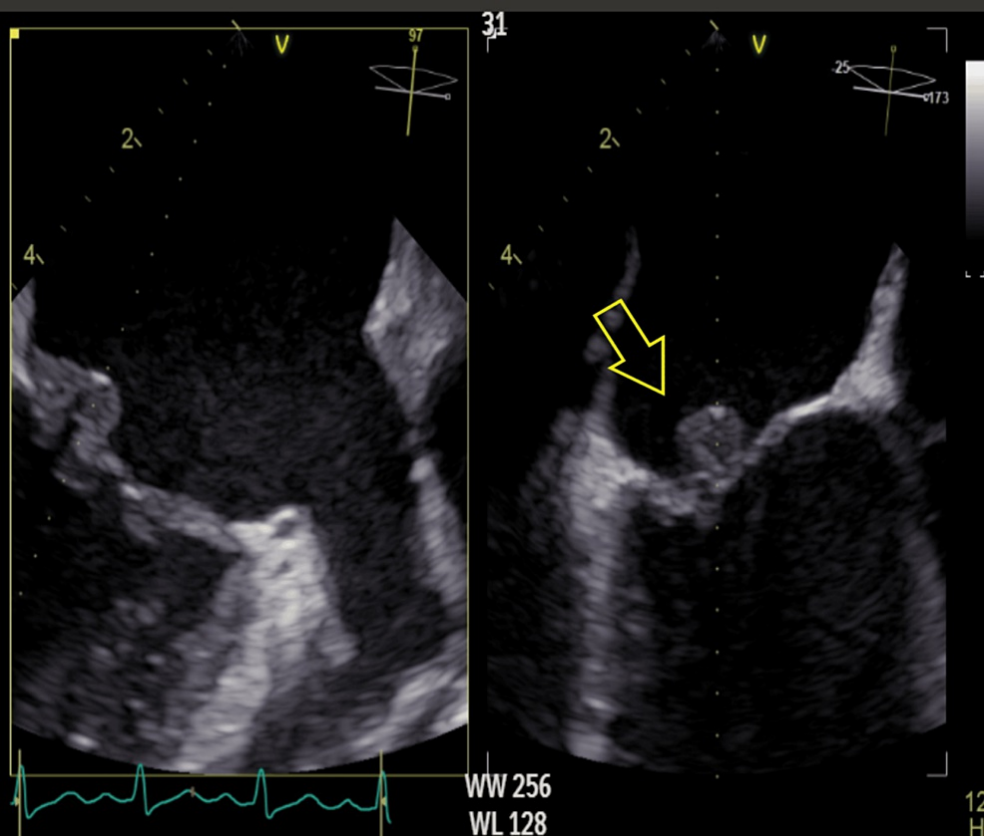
Transesophageal echocardiography shows a bulky thrombus/mass on the posterior mitral valve leaflet measuring 8 x 18 mm
